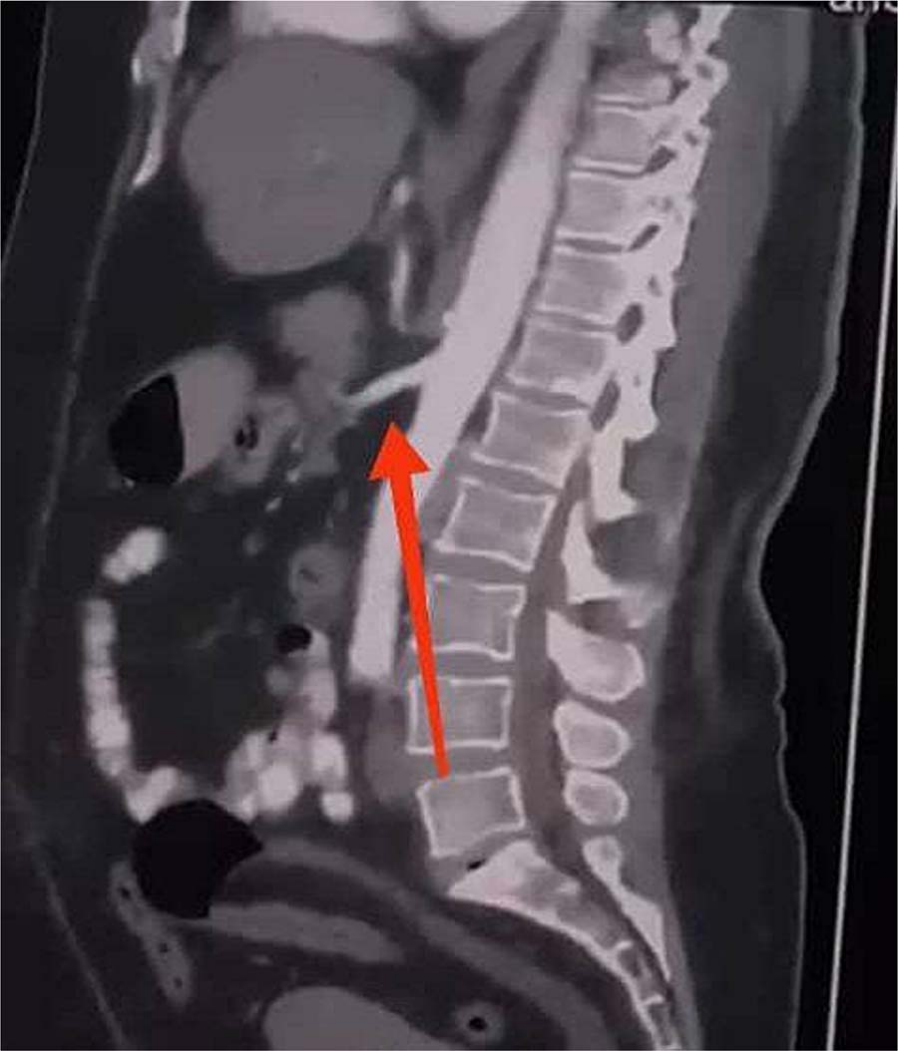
Sagittal CT scan showing a retromesenteric course of the duodenum (indicated by an arrow).
